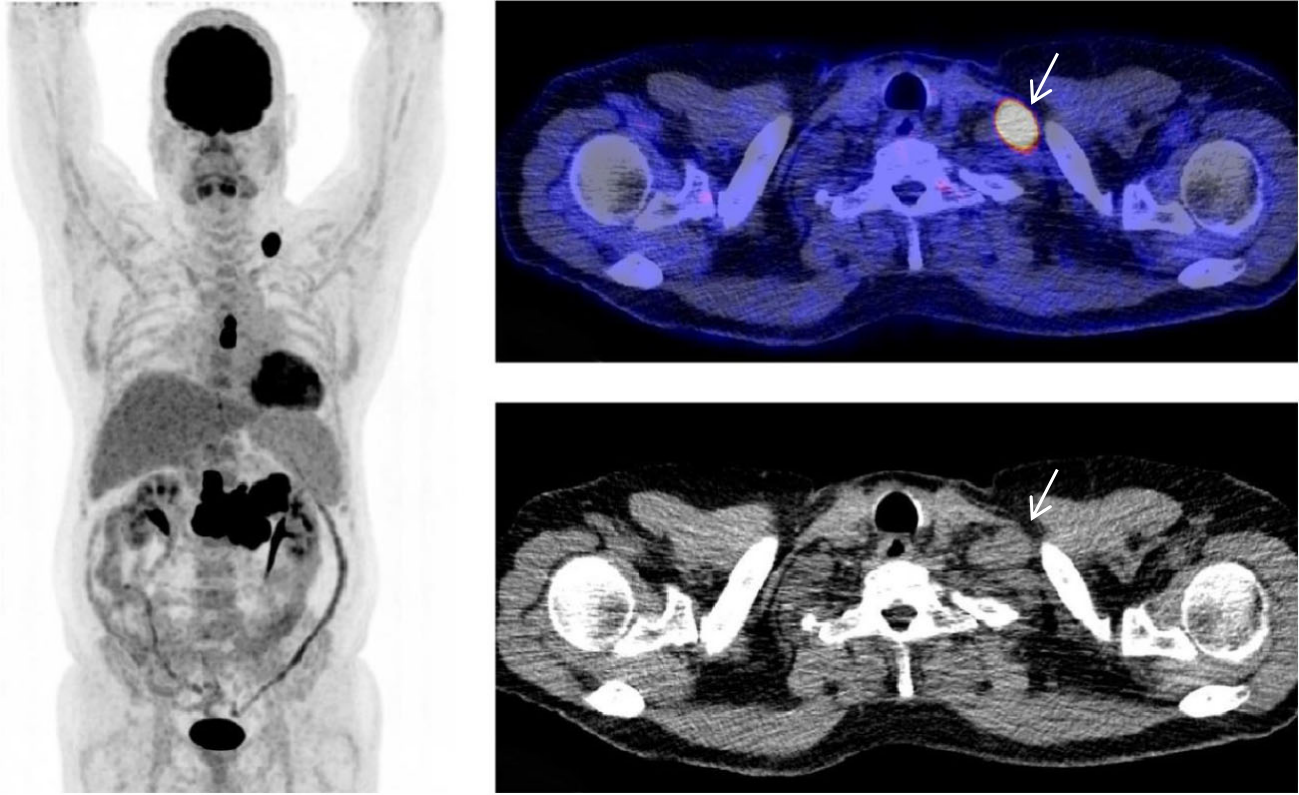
A 49-year-old male presented with low LDH levels (251 U/l) and low EBV DNA (1010 copies/ml) load, 6 years after lung transplantation. FDG-PET/CT was requested after palpable lymphadenopathy was clinically detected. Maximum intensity projection FDG-PET image shows metabolically active supraclavicular and mediastinal lymph nodes, and a large confluent abdominal lesion. Axial fused PET/CT (top right) and CT (bottom right) show the metabolically active supraclavicular lymph node, which proved to be monomorphic PTLD after biopsy
